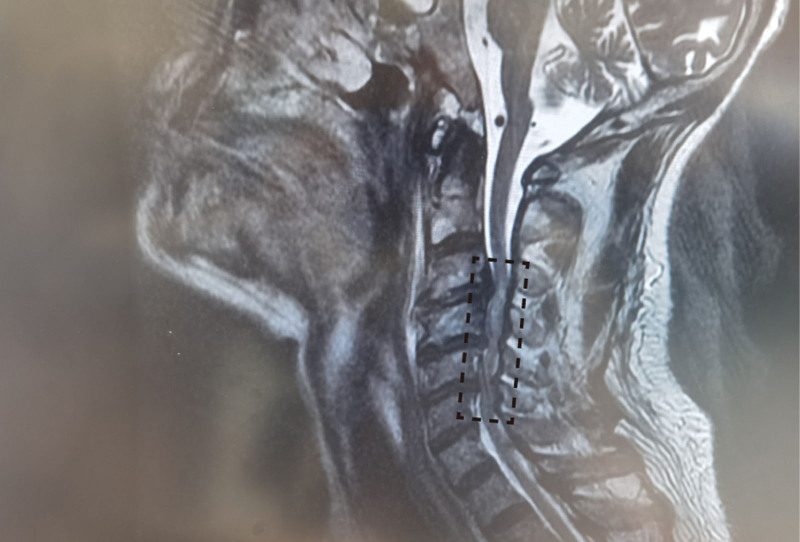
The image of cervical spine MRI. The patient showed C2/3, C3/4, C4/5, and C5/6 intervertebral disc herniation with secondary spinal stenosis (black dotted frame), accompanied by spinal cord compression and edema. MRI = magnetic resonance imaging.
